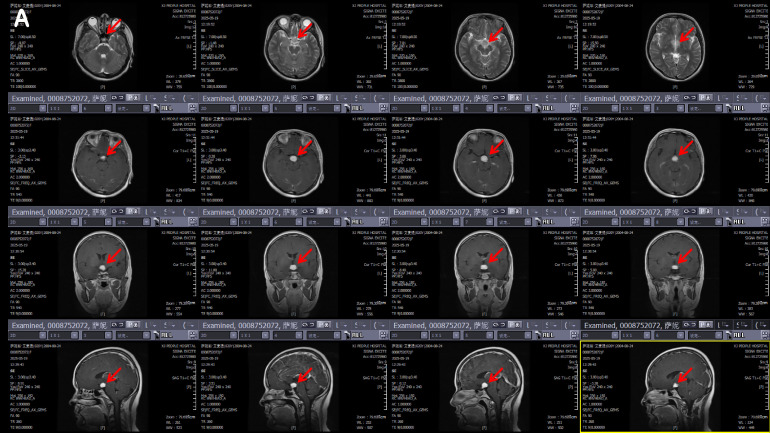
Preoperative cranial MRI demonstrating a space-occupying lesion in the hypothalamic–pituitary region.
